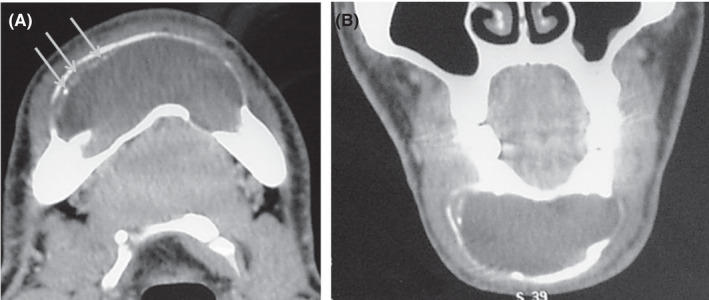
A, CT scan axial slice in narrow window showing the presence of a mixed image deforming the external and internal cortices which are thinned, with the presence of fine intra‐lesional calcifications lining the wall. B, A CT scan coronal slice showing the presence of fine intra‐lesional calcifications
